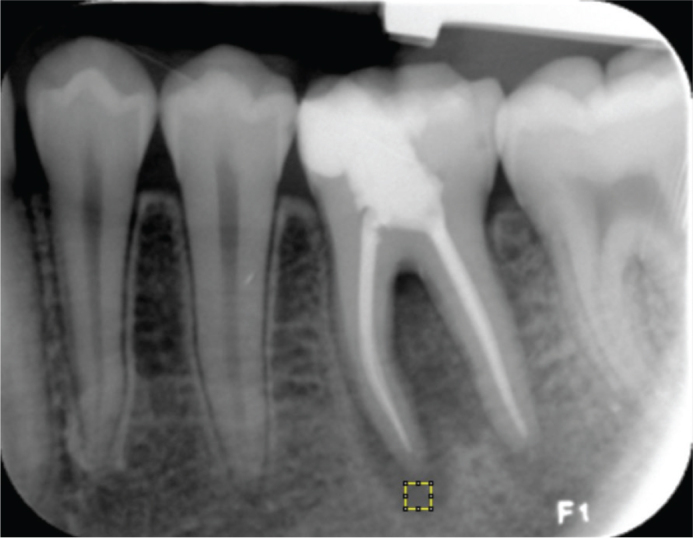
Determination of the ROI in a periapical radiograph. The yellow square shows the ROI.
